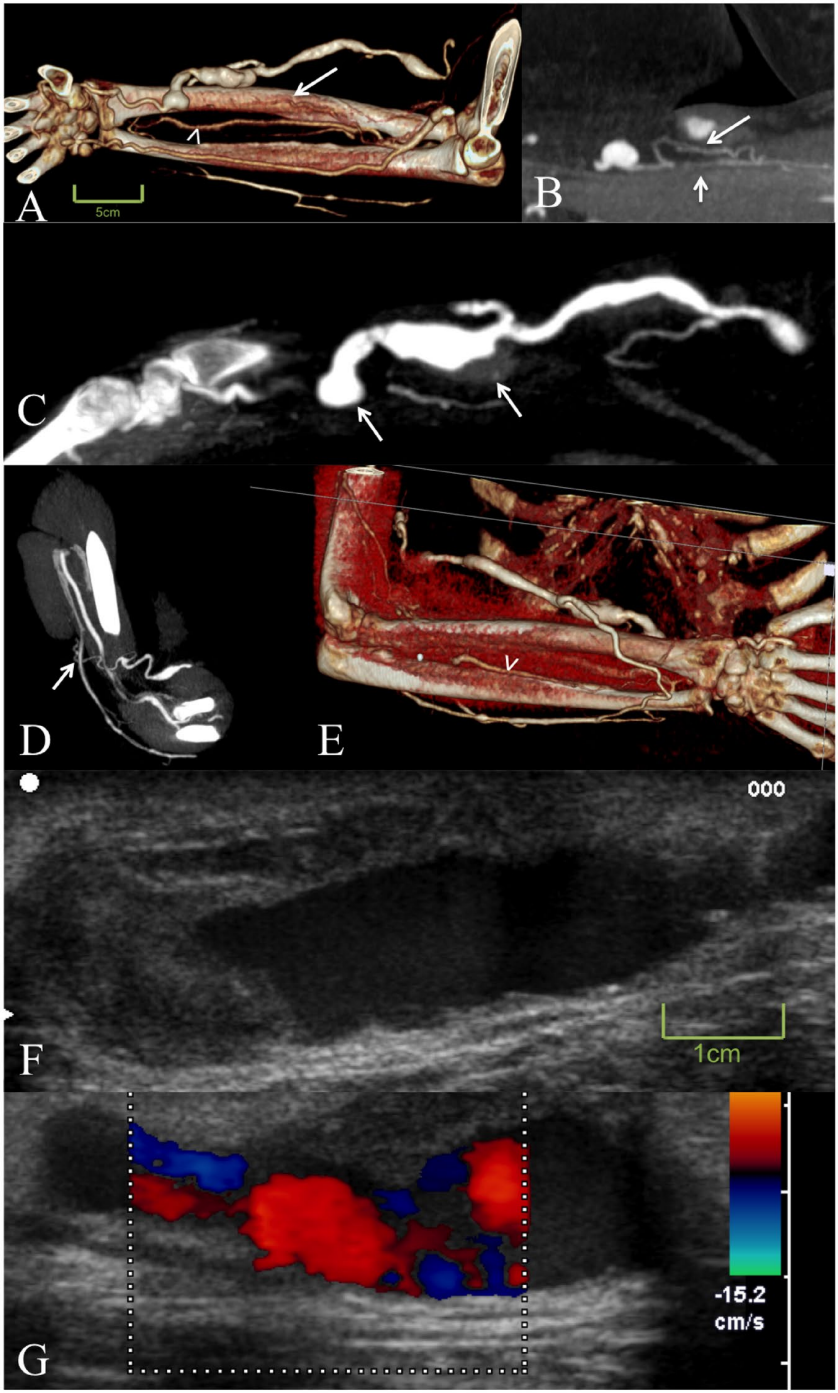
Time-resolved dynamic CT angiography of a 57-year-old female patient with a complex failing arterio-venous forearm fistula. Image (A) shows only marginal contrast of the afferent radial artery with a stenosis 2 cm proximal to the arterio-venous forearm fistula and a collateral artery as a sign for a long term stenosis (arrows in B). Image (A) also demonstrates a very strong anterior interosseous artery (arrow head in A and E) also indicating a chronic stenosis. Further, the study revealed two venous aneurysms with a wall adherent thrombus directly located after the fistula (arrows in C). The venous return revealed only drainage over the deep perforator venous system without a connection to the cephalic vein (arrow in D). The main venous return is depicted in image (E) via a dilated superficial vein to the basilica vein. Duplex ultrasound images in F and G demonstrate the partially thrombotic aneurysm but do not reveal the stenosis proximal to the fistula, as well as the insufficient venous backflow to the cephalic vein.
